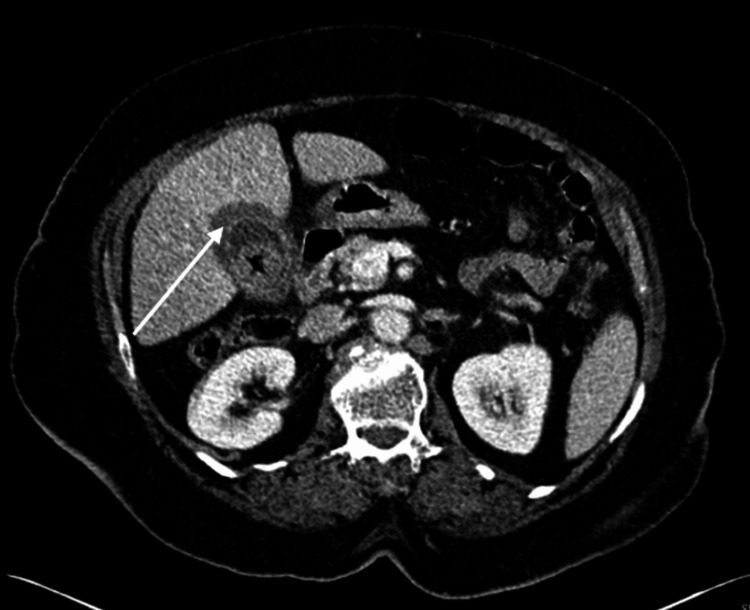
Computed tomography image shows gallstone, gallbladder thickening, and pericholecystic fluid Arrow indicates gallbladder thickening
